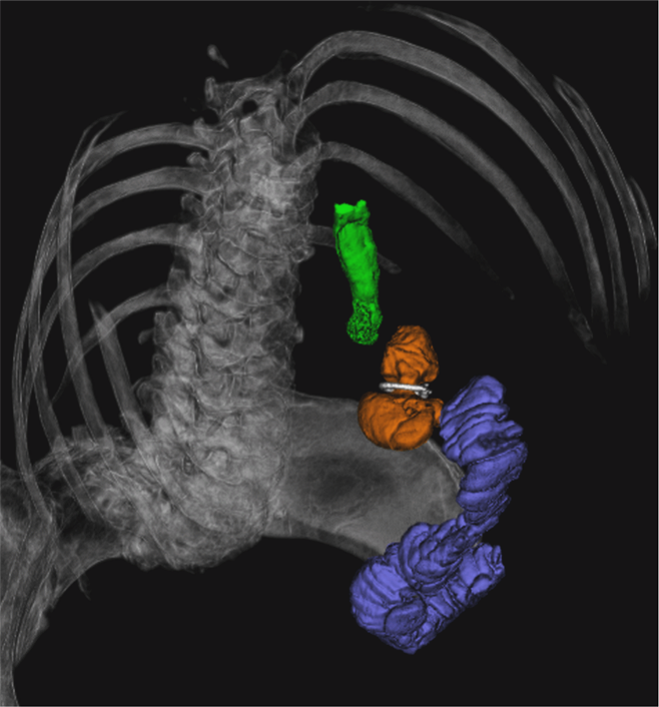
The banded gastric bypass using the GaBP™-ring. 3D-reconstruction of abdominal multi slice computed tomography: green: oesophagus, orange: gastric pouch, white: GaBP ring, blue: jejunum.
